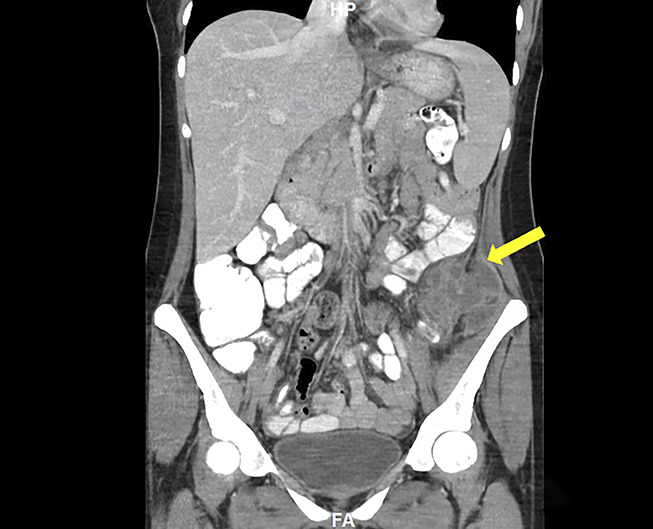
Abdominal and pelvic CT with oral and IV contrast (axial view) revealed mucosal thickening of a short segment of the descending colon measuring 5 cm in length (yellow arrow), causing severe lumen narrowing and partial obstruction of indeterminate etiology. CT, computed tomography; IV, intravenous.
